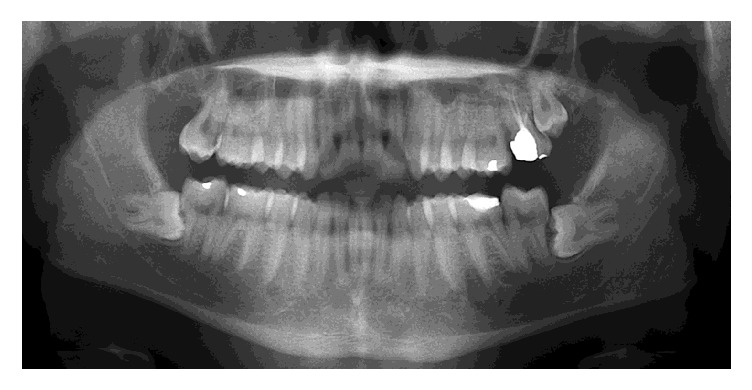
Orthopantomograph shows a well-circumscribed radiolucent mass attached to the lateral root surface of impacted permanent left mandibular first molar.
